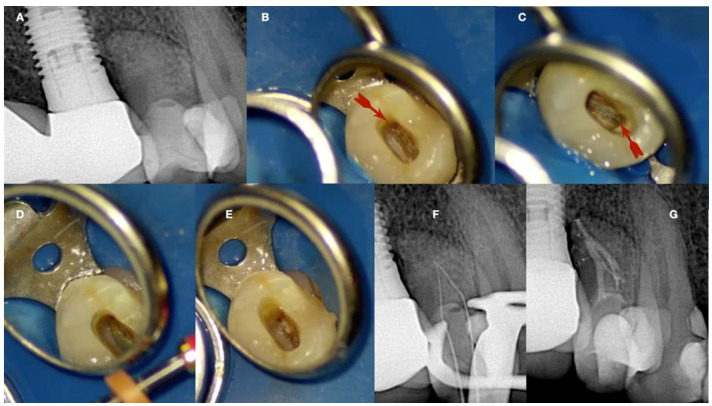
Calcified maxillary premolar negotiation (A). Preoperative radiograph of a calcified maxillary premolar associated with apical periodontitis (B). Clinical view of the palatal calcified canal orifice (red arrow) (C). Clinical view of the buccal calcified canal orifice after chasing the pulp floor developmental line to its far end (red arrow) (D). Clinical view of the EDM file buckling resistance activation test negotiation (E,F). Clinical and radiographic images of the calcified canal negotiation, (G) postoperative radiograph of the calcified maxillary premolar treatment (clinical images and radiographs are courtesy of Dr. Chaniotis Antonis).
